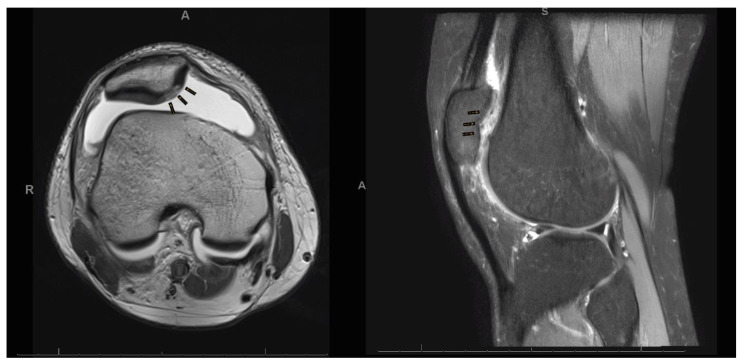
MRI of a focal chondral defect of the patella.
